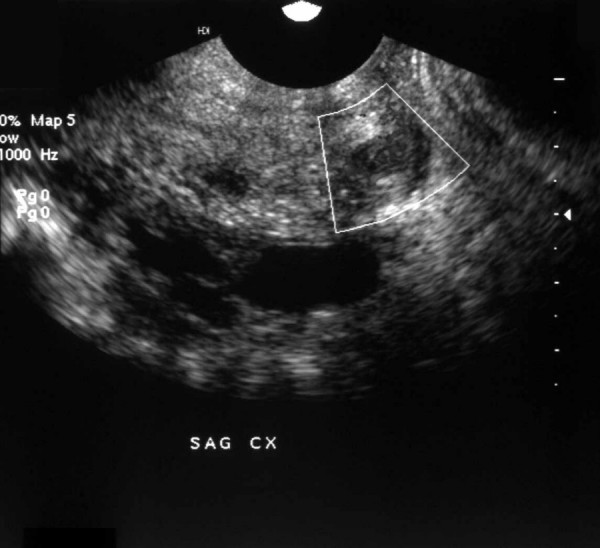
Ultrasonographic appearance of the cervical mass showing it to be deceptively circumscribed
